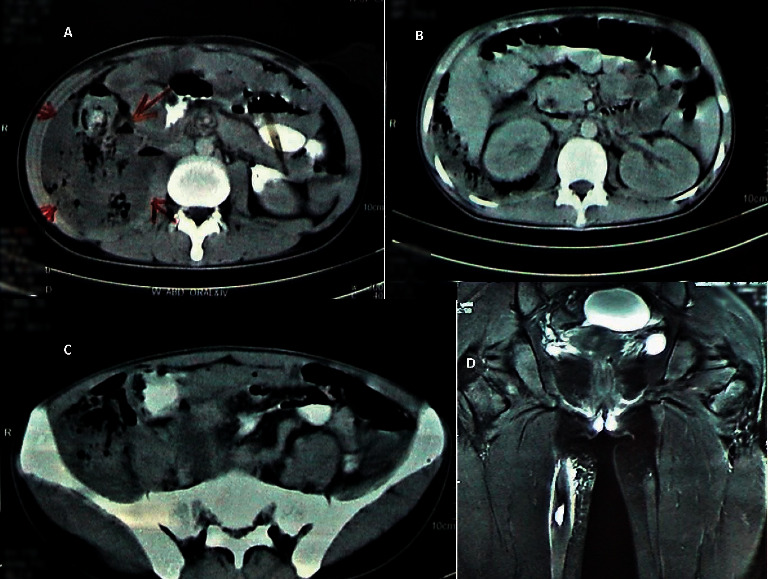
CT scan abdomen with oral contrast showed a large retroperitoneal collection with multiple air lucencies displacing ascending colon and caecum medially. (a) Discontinuity in the posterior wall of ascending colon with a large collection having internal air lucencies, (b) collection with internal air lucencies seen in the right pararenal and posterior perihepatic soft tissue planes, (c) retroperitoneal collection seen tracking into the right iliac fossa, and (d) elongated collection and surrounding soft tissue oedema seen along hamstring muscles of the right thigh.
